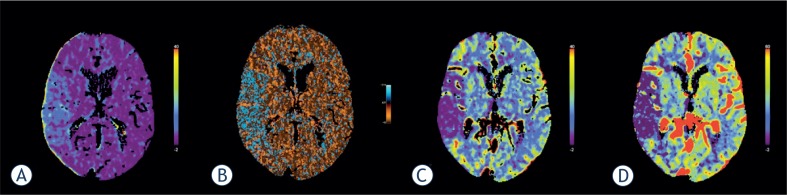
Perfusion computed tomography in acute stroke. Parametric maps show increased blood-brain barrier permeability values (A, B) in the right middle cerebral artery territory. The main advantage of Patlak’s analysis is its conceptual simplicity (A). On the other hand, distributed parameter model takes the tracer concentration gradients within vasculature into account and may allow more complete analysis of kinetic parameters (B). The delineation of ischaemic area is clearly recognized on blood flow (C) and blood volume (D) parametric maps.
